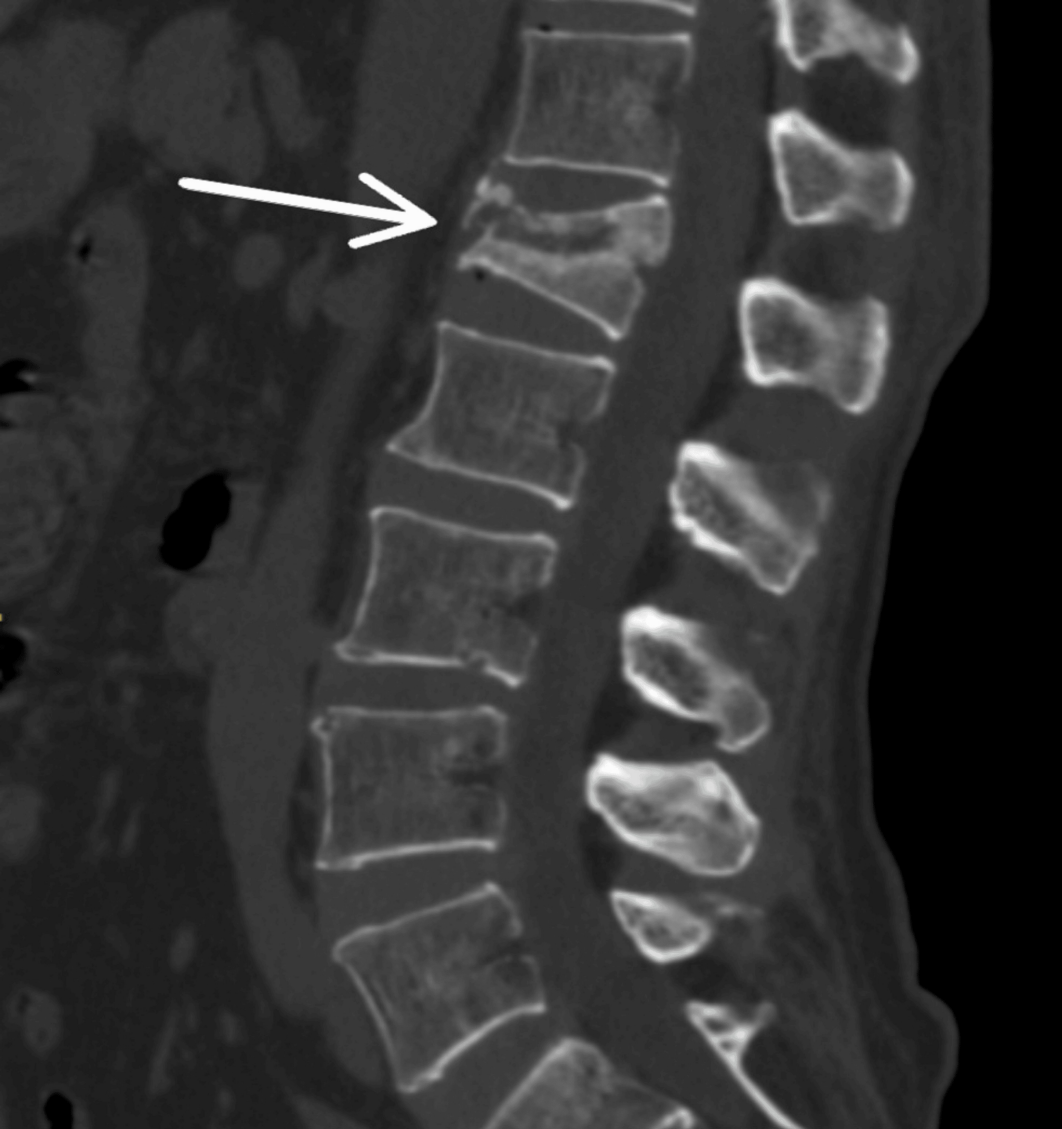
Lateral CT scan view showing L1 vertebral fracture.
